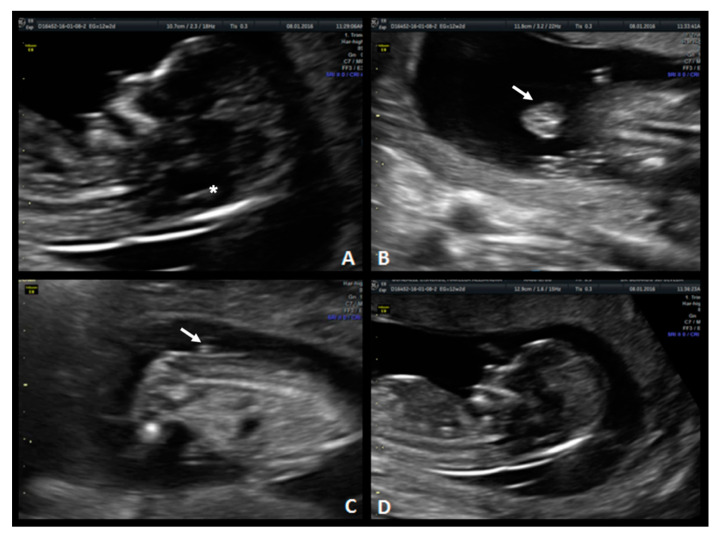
Case 4. (A) Mid-sagittal plane of the fetal face demonstrates compression of the fourth ventricle with no visible IT (*). (B) Ultrasound view of the lower back with a lumbar spine defect (white arrow). (C) Sagittal plane of the spine shows the neural tube defect (white arrow). (D) No evident IT.
